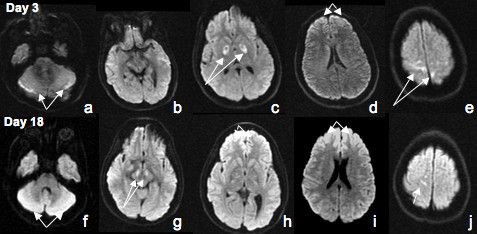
Top row: DWI sequences of MRI brain obtained at day #3 after admission showing abnormal signal in the cerebellum (a), globus pallidus bilaterally (c), motor cortex (d and e). Bottom row: DWI sequences of MRI brain at day #18 after admission showing more attenuated and confluent cerebellar lesions (f), new hyperintensities in the cerebral peduncles (g), and frontal cortex (h and i), persistent smaller lesions in the motor cortex (j).
